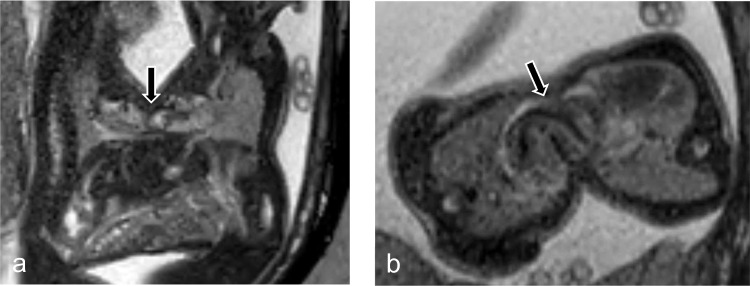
Fetal MRI of thoracopagus at gestational age 24 weeks, 6 days. a BTFE sagittal and b BTFE axial to the conjoined torso both show a shared, complex multichambered heart (*black arrows*). *BTFE*, balanced turbo-field echo
